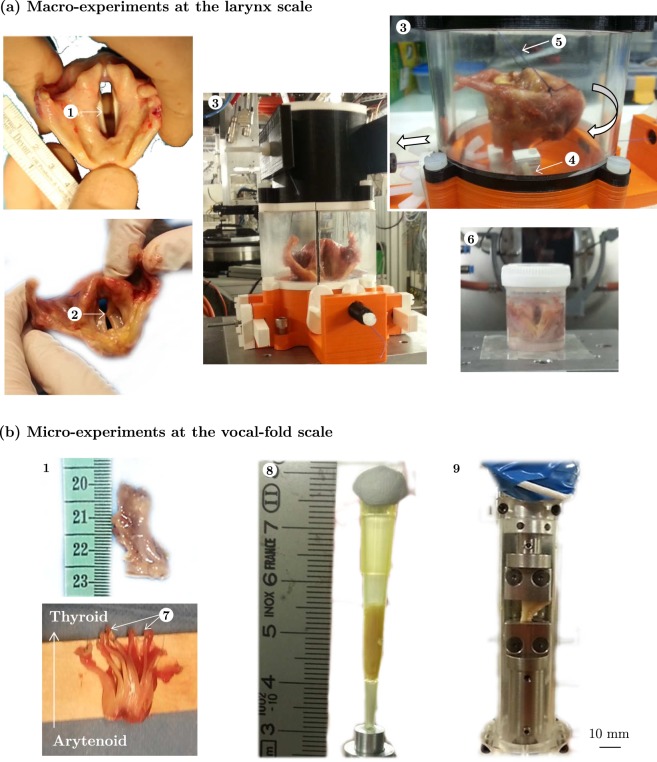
Experimental set-ups developed for X-ray microtomography characterisation of the vocal-fold tissues (a) within the preserved laryngeal structure and (b) once dissected. ① Vocal fold, ② Stitch between vocal processes, ③ Macro-mechanical set-up, ④ Wooden pike, ⑤ Wires blocking thyroid’s branches, ⑥ Set-up for sample L_9_, ⑦ Dissection procedures for samples L_*i*_-S_*j*_, ⑧ Pilot set-up, ⑨ Micro-mechanical tension device.
